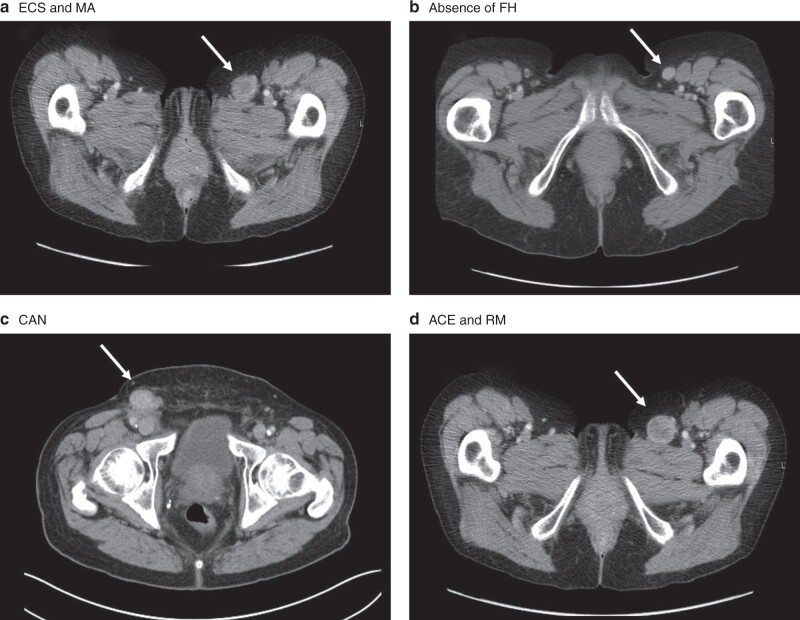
Six CT characteristics of lymph nodes used to indicate presence of lymph node metastases in melanoma a Extracapsular spread (ECS) and minimum axis (MA); b absence of fatty hilum (FH); c asymmetrical cortical nodule (CAN); d abnormal contrast enhancement (ACE) and rounded morphology (RM).
